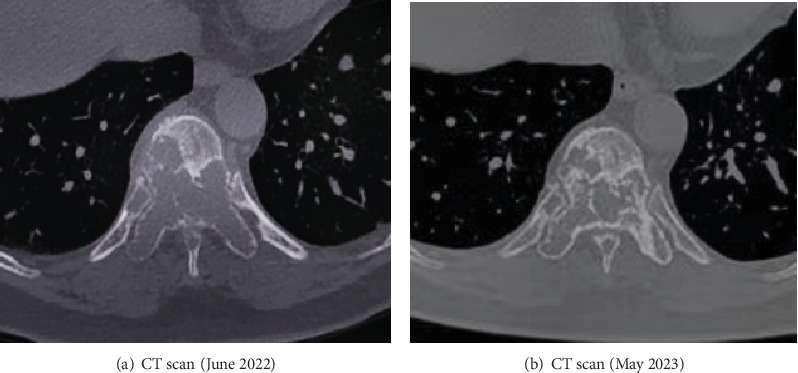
Comparison of CT bone window at the same level from June 2022 and May 2023. In June 2022, (a) CT bone window showed local bone trabecular destruction of the T10 vertebral body, accessories and adjacent ribs, and slight swelling changes in local bone structure. (b) CT bone window from May 2023 revealed bone density deposits in the damaged area of the original bone trabecula.
